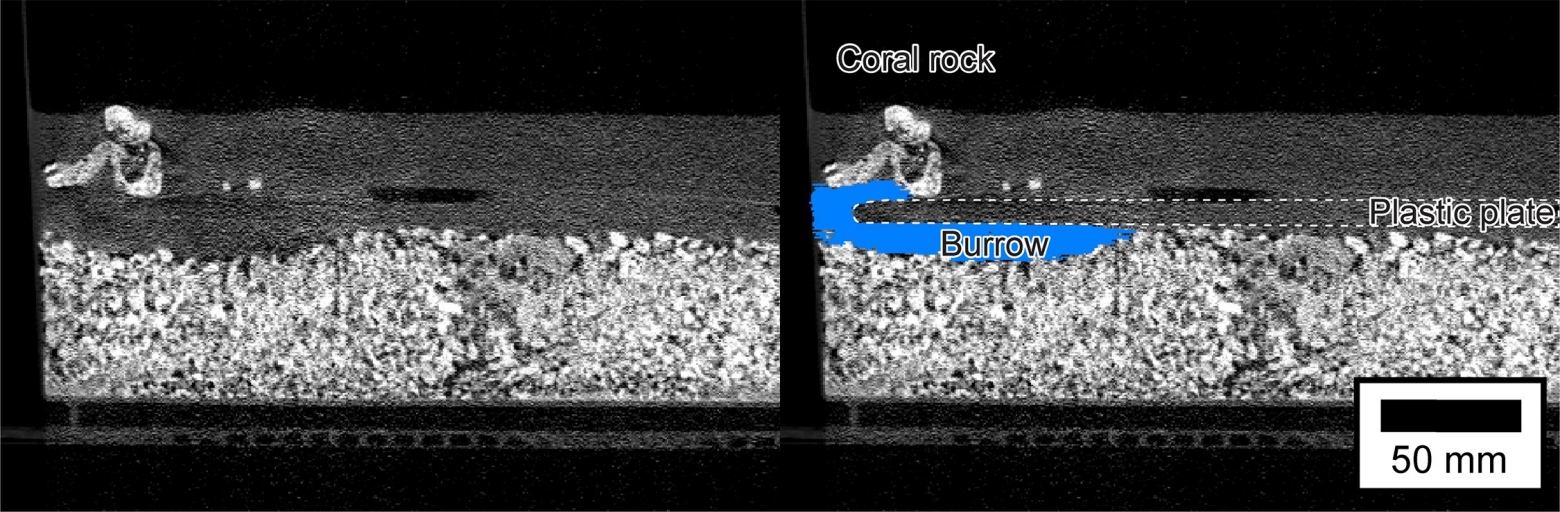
Cross section of an *Alpheus bellulus* burrow imaged by X-ray computed tomography. The blue shaded region in the right-hand panel shows the location of the burrow.
